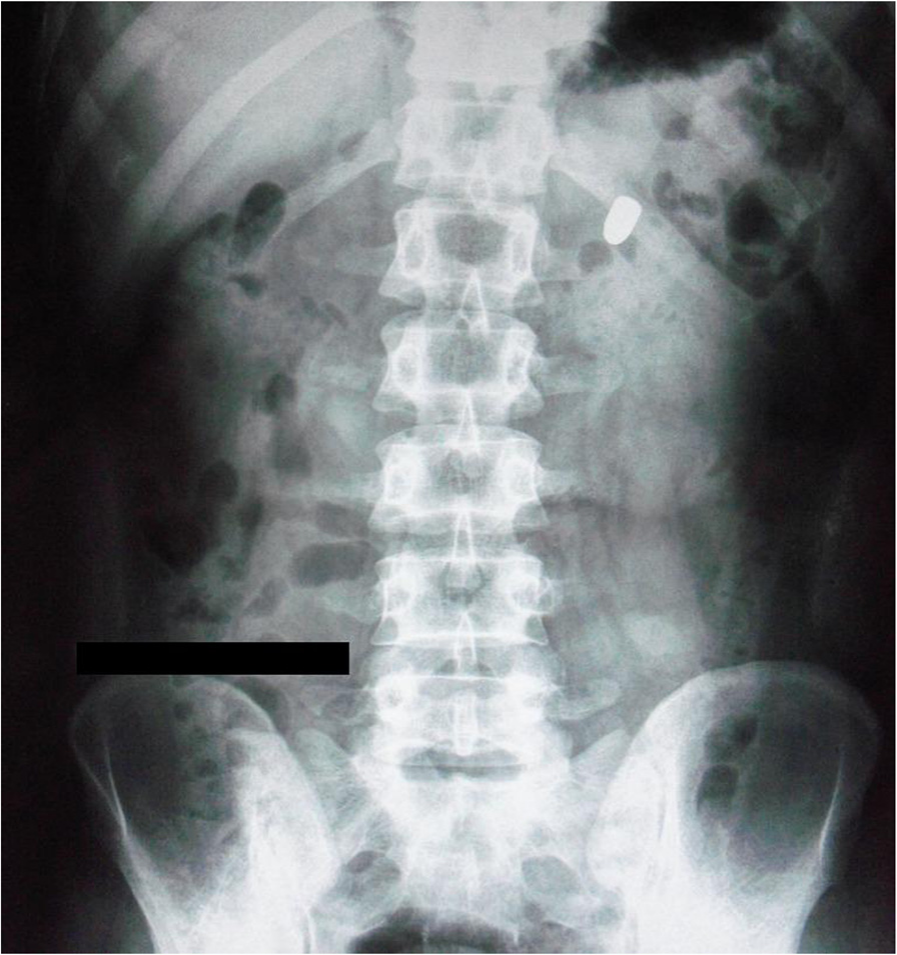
Erect abdominal radiograph. Radiograph showing bullet in the upper left quadrant of the abdomen.
